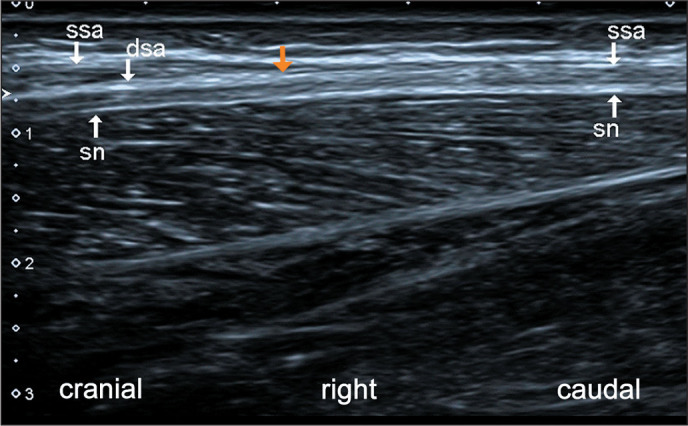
Longitudinal ultrasonography of the right calf, showing the sural nerve
